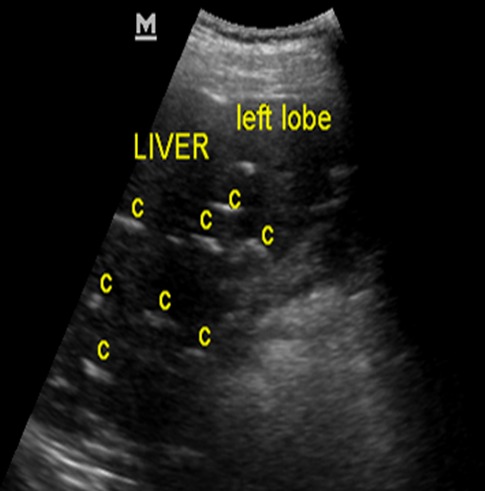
Abdominal ultrasonogram of case 2 at the level of the liver showing multiple brightly echogenic lesions (C) casting posterior acoustic shadows in the liver parenchyma, distorting its architecture
